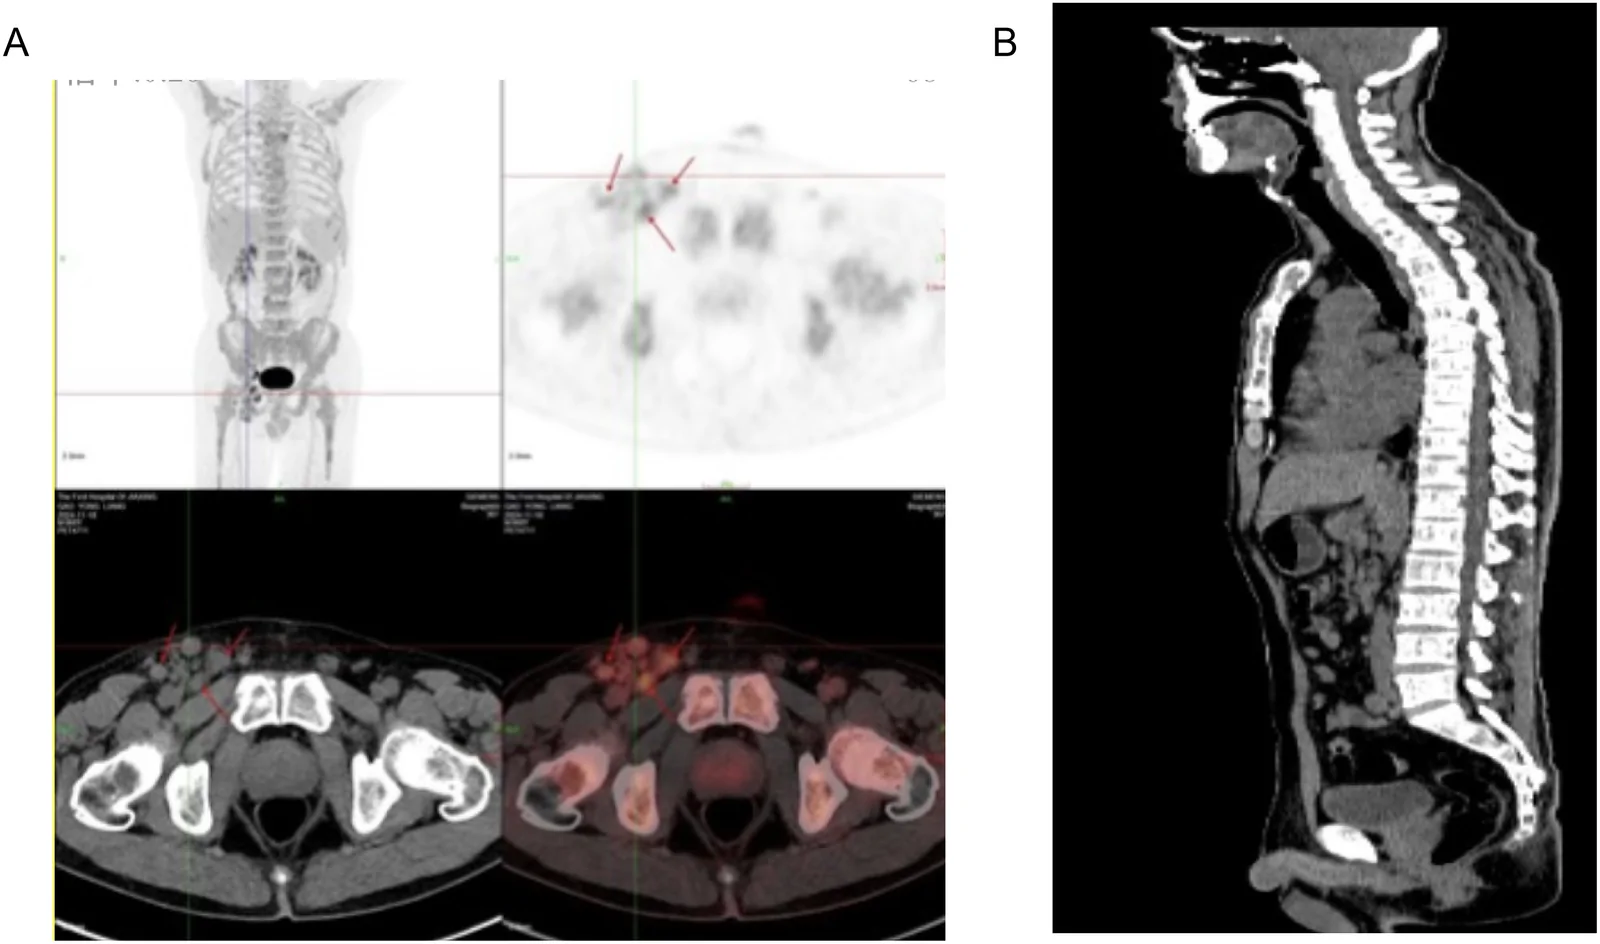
PET-CT showed localized thickening of the right scrotal skin with mildly increased patchy FDG metabolism, suggestive of a malignant skin lesion; multiple enlarged lymph nodes with increased FDG metabolism in the right inguinal region, right iliac vessel area, bilateral pelvic walls, bilateral common iliac arteries, para-aortic region, and left supraclavicular area, and multiple osteolytic lesions within the visualized field (A, B).
